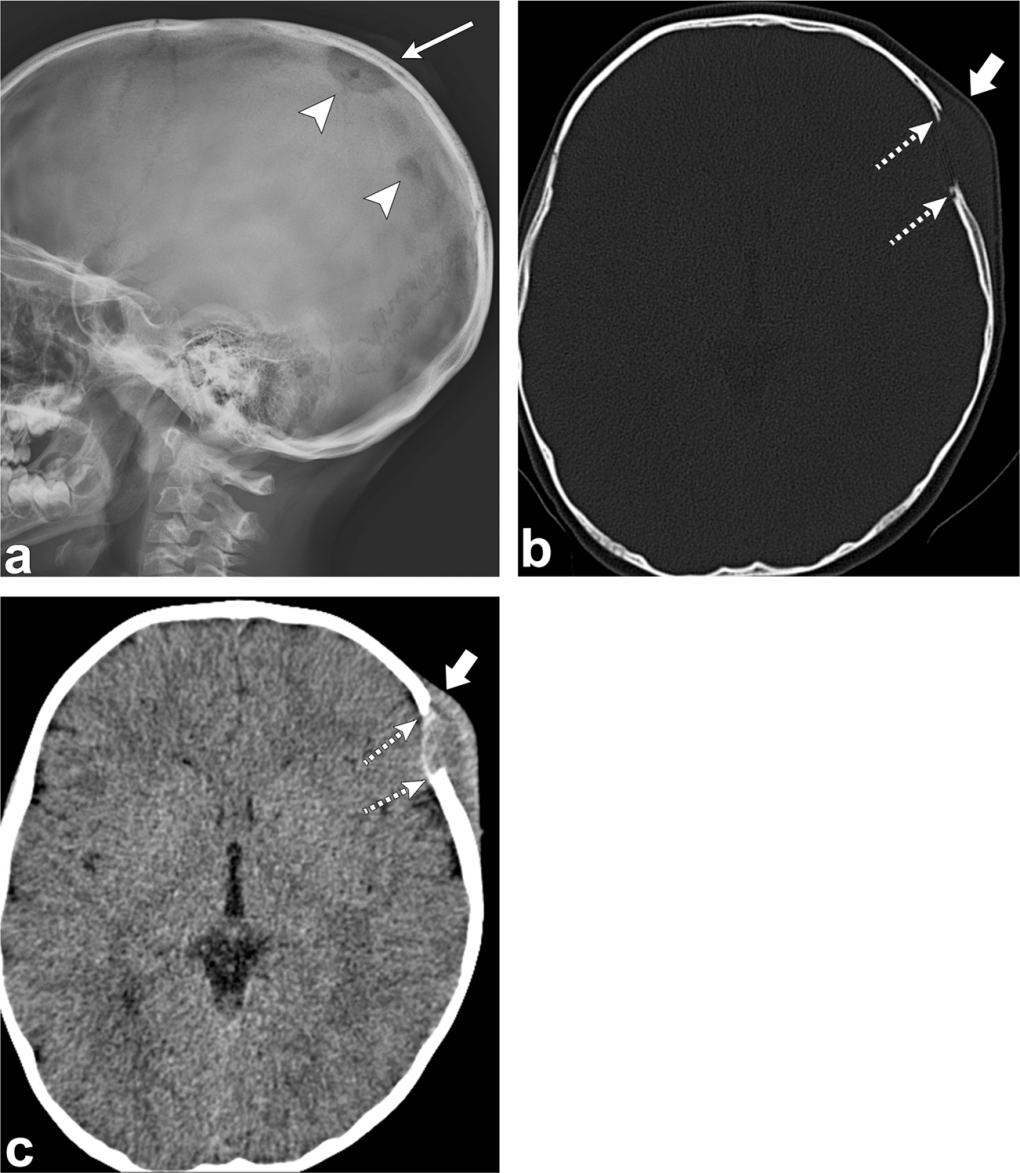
Langerhans cell histiocytosis. Sagittal skull radiograph (a) shows two lytic lesions (*arrowheads*) in the parietal skull, the largest one with an associated soft tissue component (*thin arrow*). Axial head CT images (b, c) in the bone and soft tissue windows in the same patient demonstrate an osseous destructive lesion in the left frontal calvarium with bevelled edges (*dashed arrows*) and a soft tissue component (*thick arrows*); pathology proven eosinophilic granuloma in a 4-year-old boy
